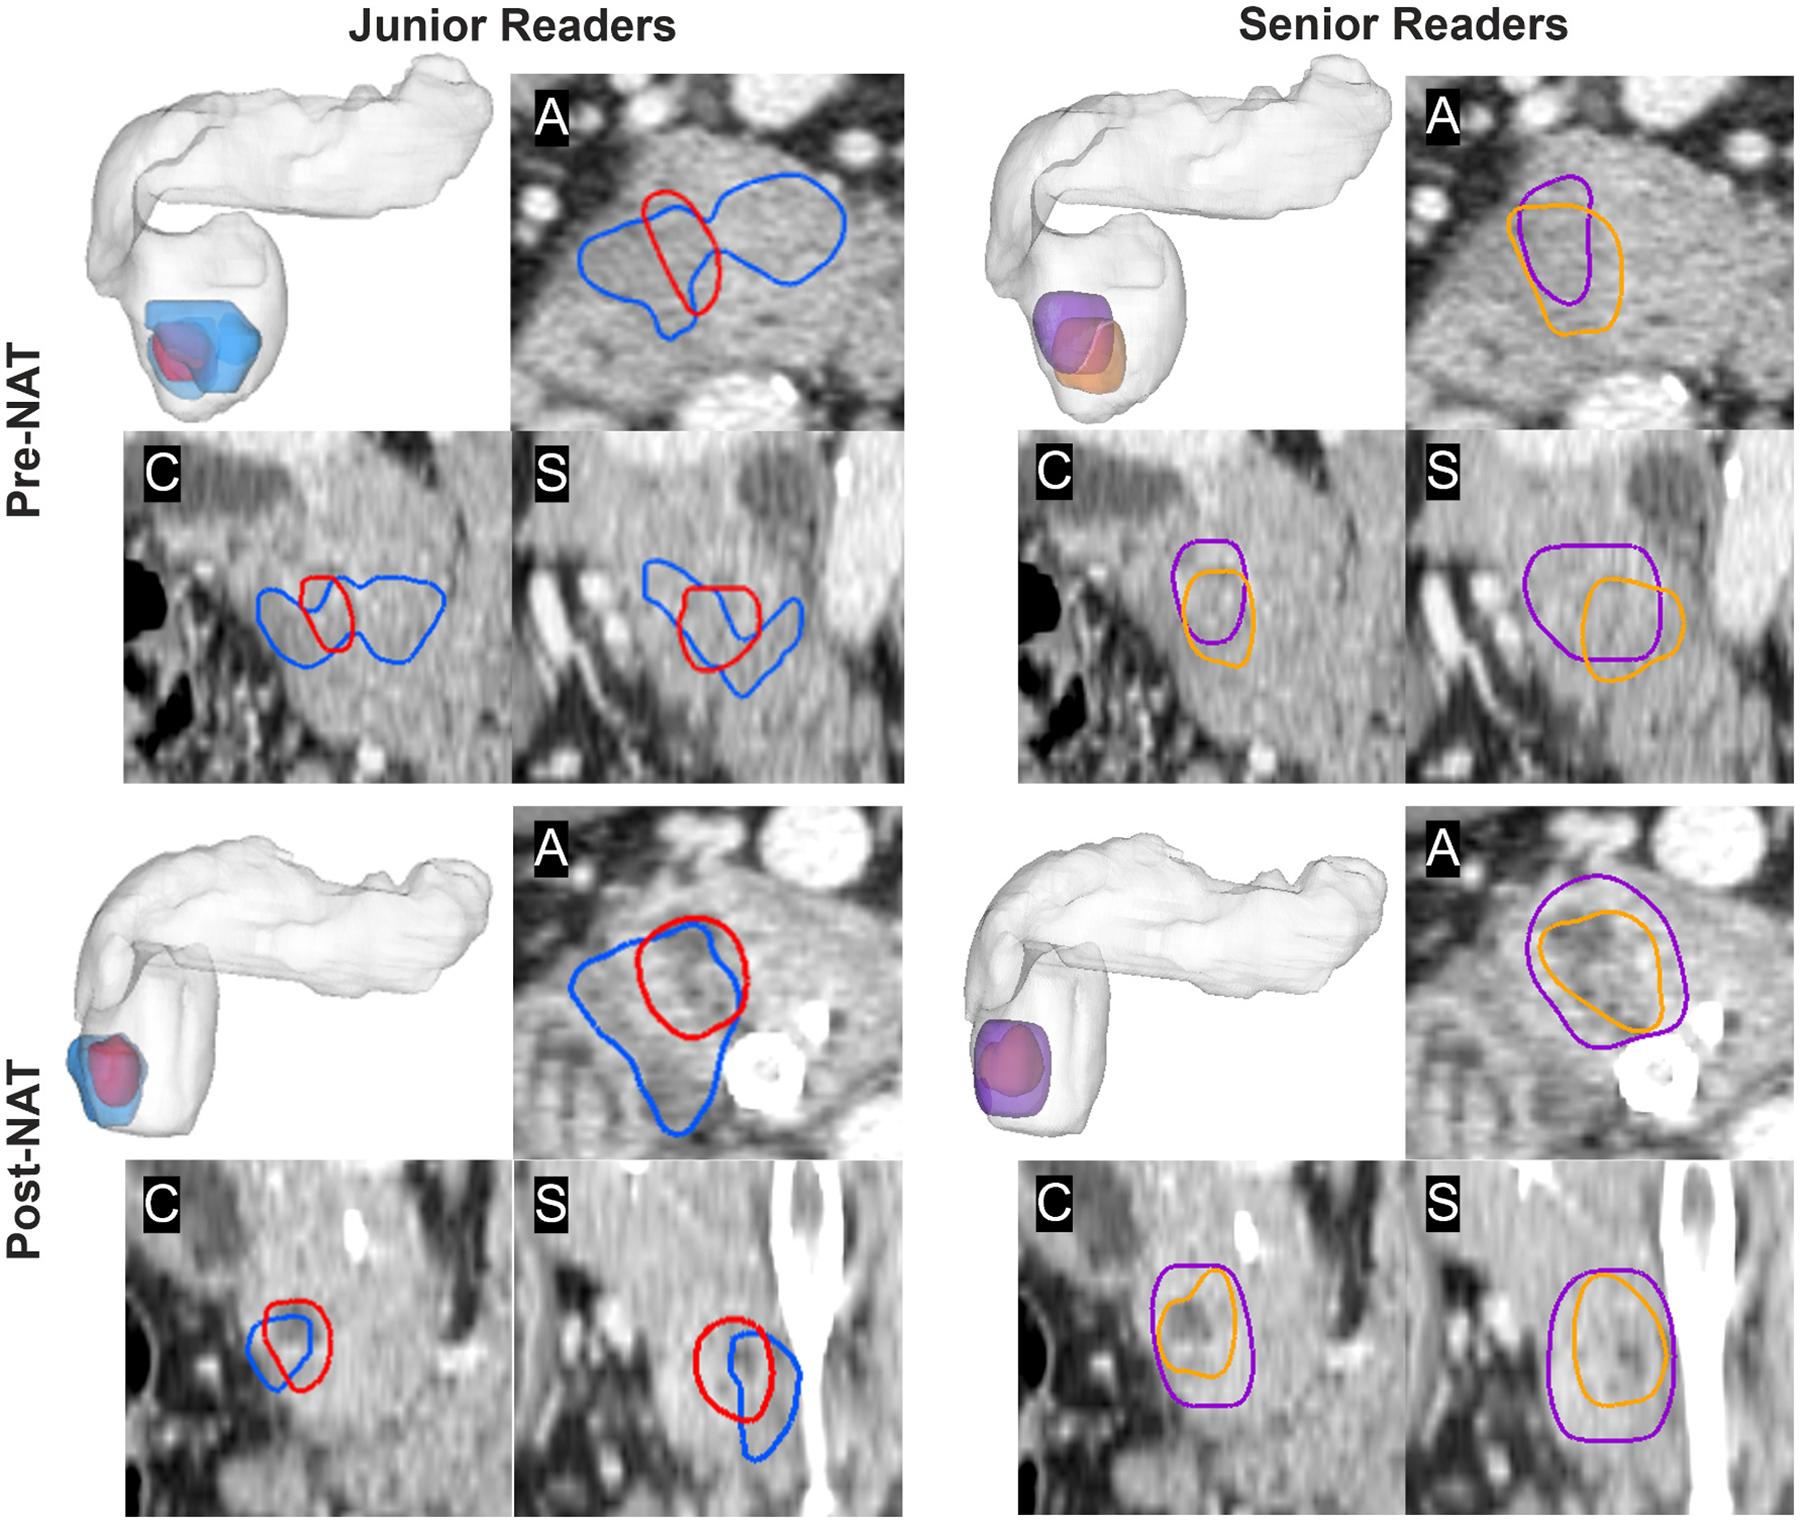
Variability of PDAC lesion segmentations among junior readers (left) and senior readers (right) on pre-NAT and post-NAT CT in one representative patient. (A) axial slice; (C) coronal slice; (S) sagittal slice.
